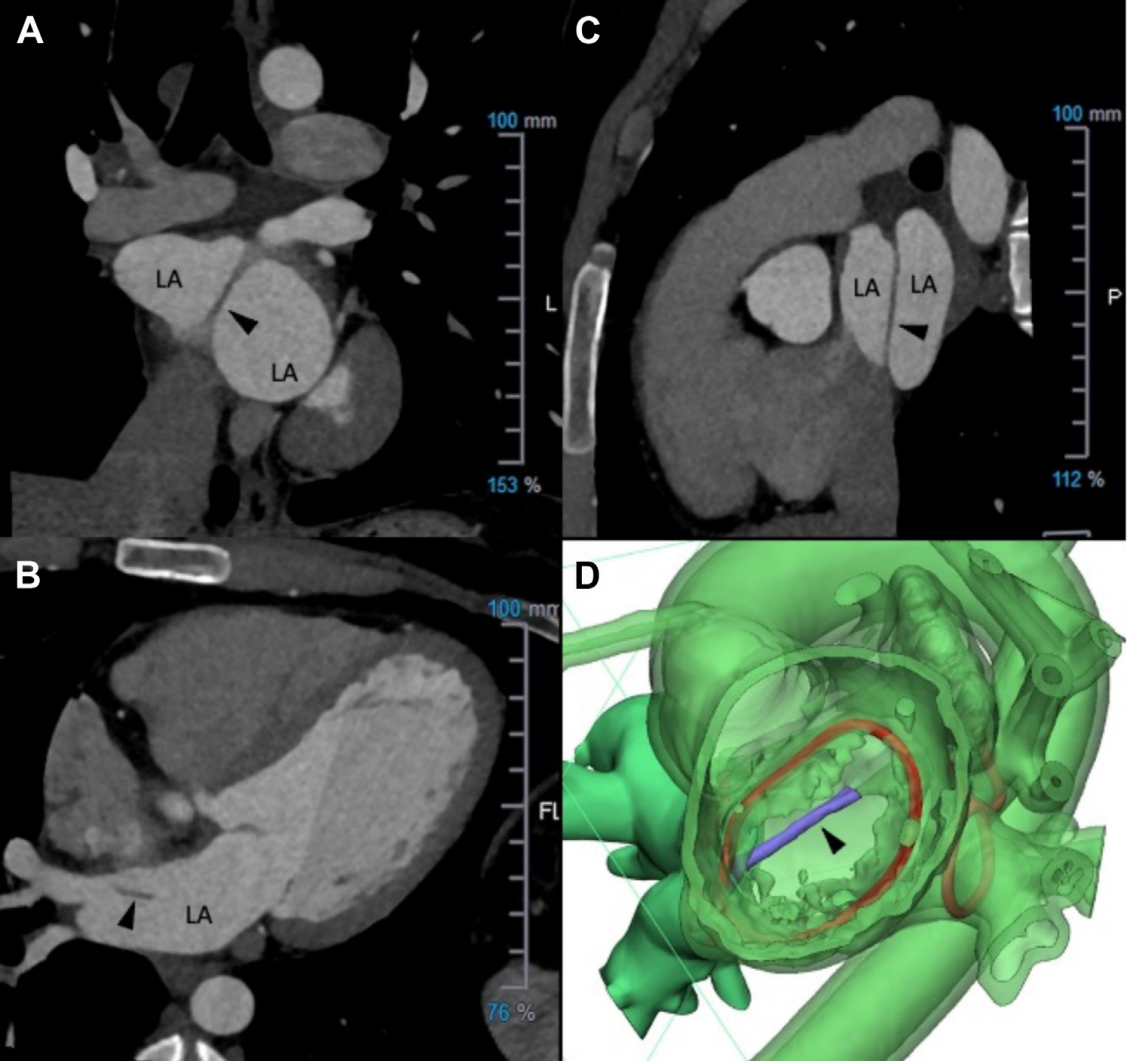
Cor Triatriatum Sinister Cardiac computed tomography image in coronal plane (A), axial plane (B), and sagittal plane (C). Apical basal view of 3-dimensional digital cardiac image of Cor triatriatum sinister (CTS) (D). Arrowheads point to a band of CTS tissue. In D, the CTS is shown in purple, and mitral valve annulus is shown in the red circle in center. LA = left atrium.
